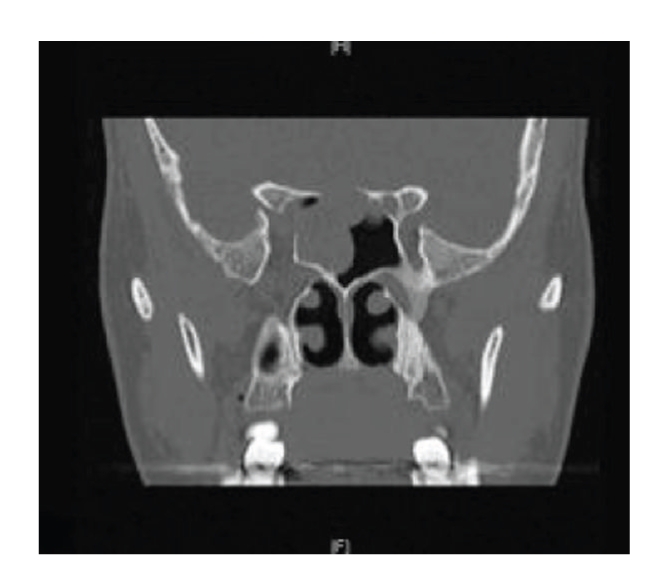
Coronal CT scan showing soft tissue mass in the sphenoid sinus with destruction of the roof of the sphenoid sinus.
